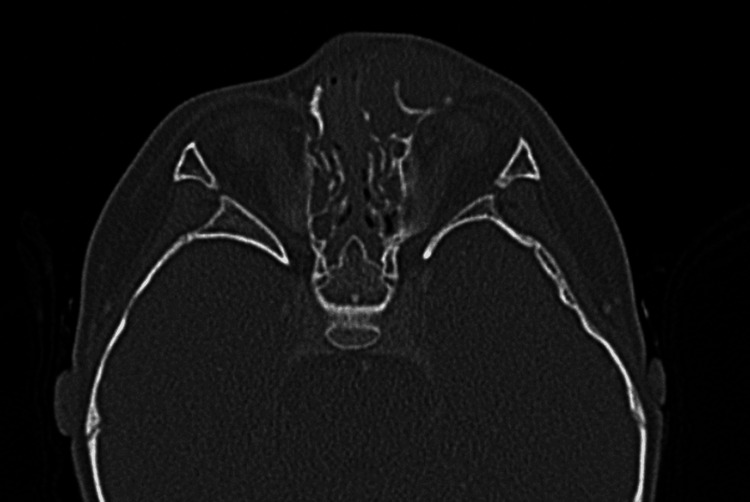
Plain computed tomography showing tumor expansion and bone destruction.
